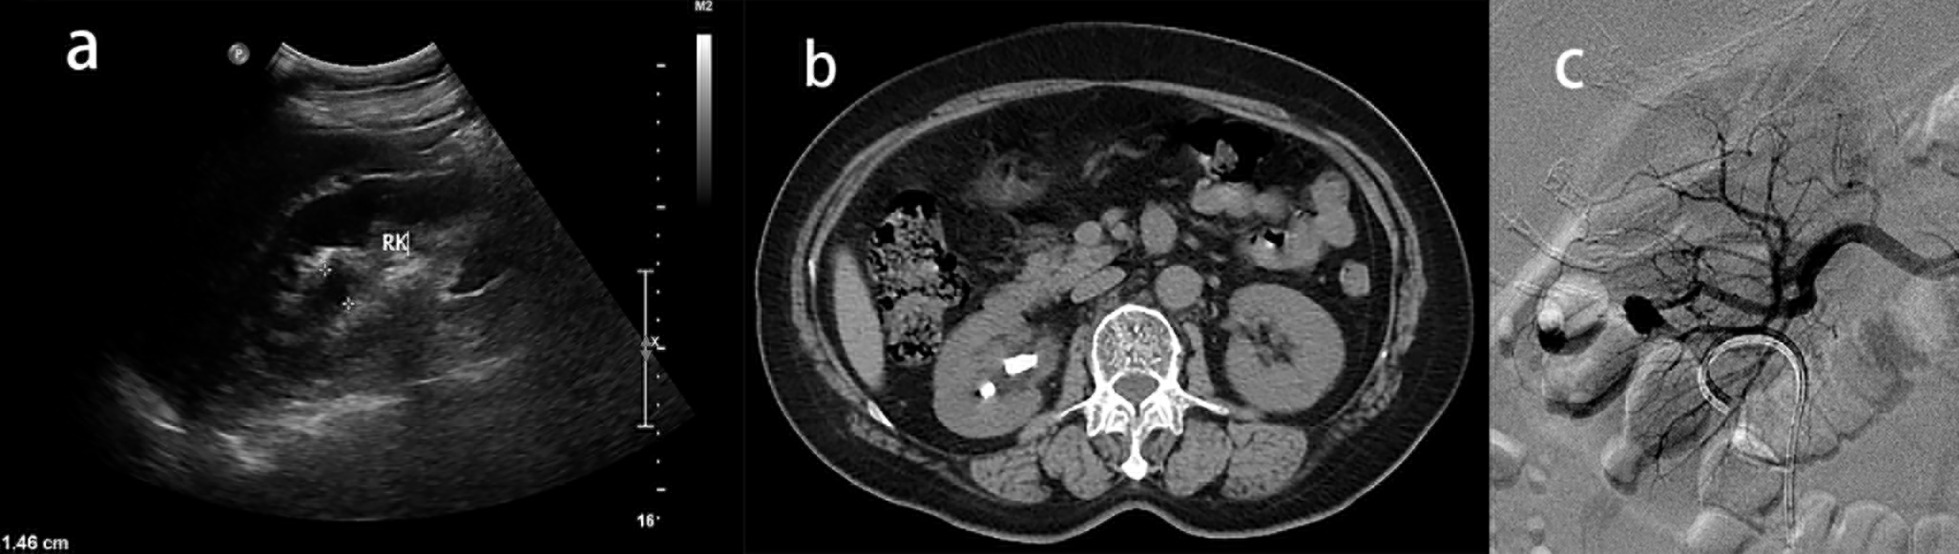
Severe complication after PCNL in 56-year-old woman who underwent CT scan and nephrosonography preoperatively. a Sonagraphic view demonstrated anteroposterior diameter (APD) of right kidney was 14.6 mm, mild hydronephrosis. b CT view of no staghorn calculi. c Superselective renal arteriogram showed extravasation in right kidney mid segmental branches
